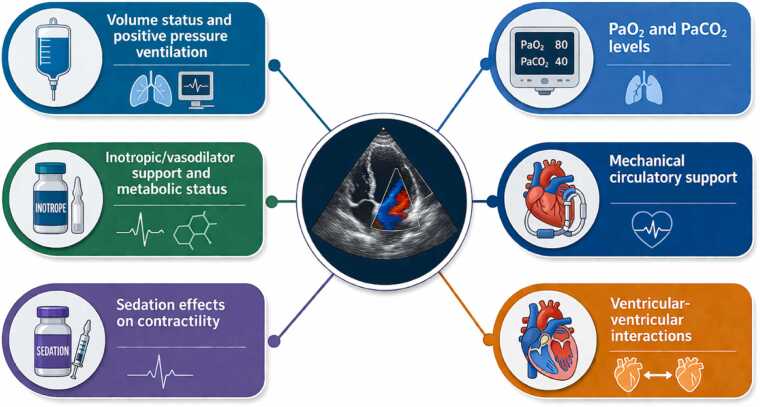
Key considerations which may influence interpretation of echocardiographic findings in ICU patients (CCE).^6^
